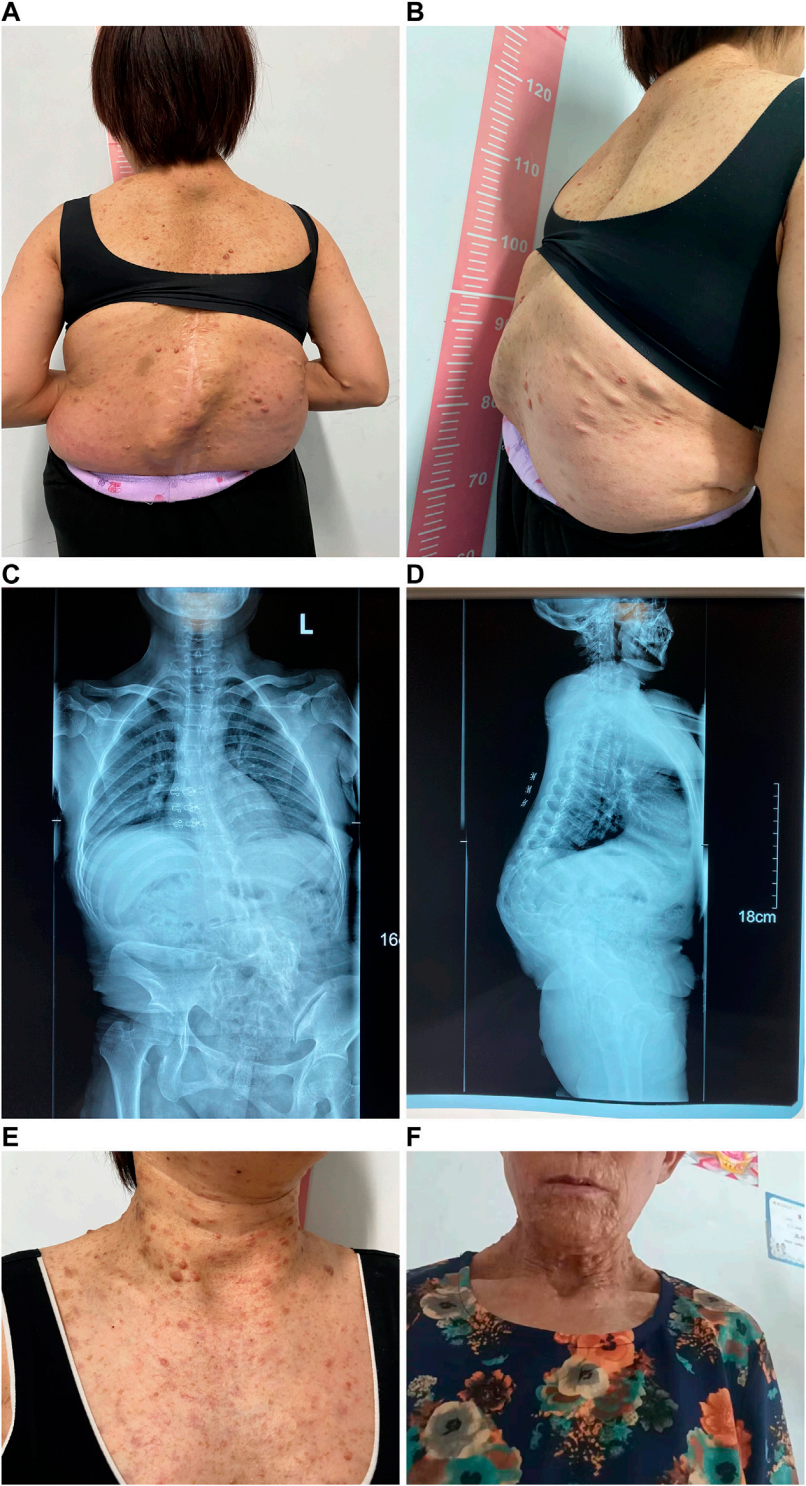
Appearance photos and X-ray images of proband’s mother. (A) Photo of the back with multiple café-au-lait macules, dermatofibroma, and severe scoliosis and kyphosis. (B) Lateral photo of the back showing severe scoliosis and kyphosis. (C) X-ray image of spinal scoliosis. (D) X-ray image of spinal scoliosis and kyphosis. (E) Appearance photo of café-au-lait macules and dermatofibroma on the neck and front chest of the proband’s mother. (F) Appearance photo of dermatofibroma around the mouth of the proband’s grandmother.
